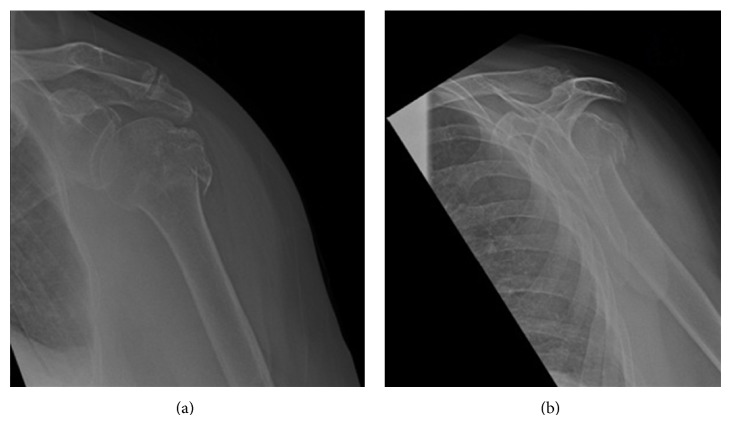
AP (a) and lateral (b) radiographs of left proximal humeral fracture.
